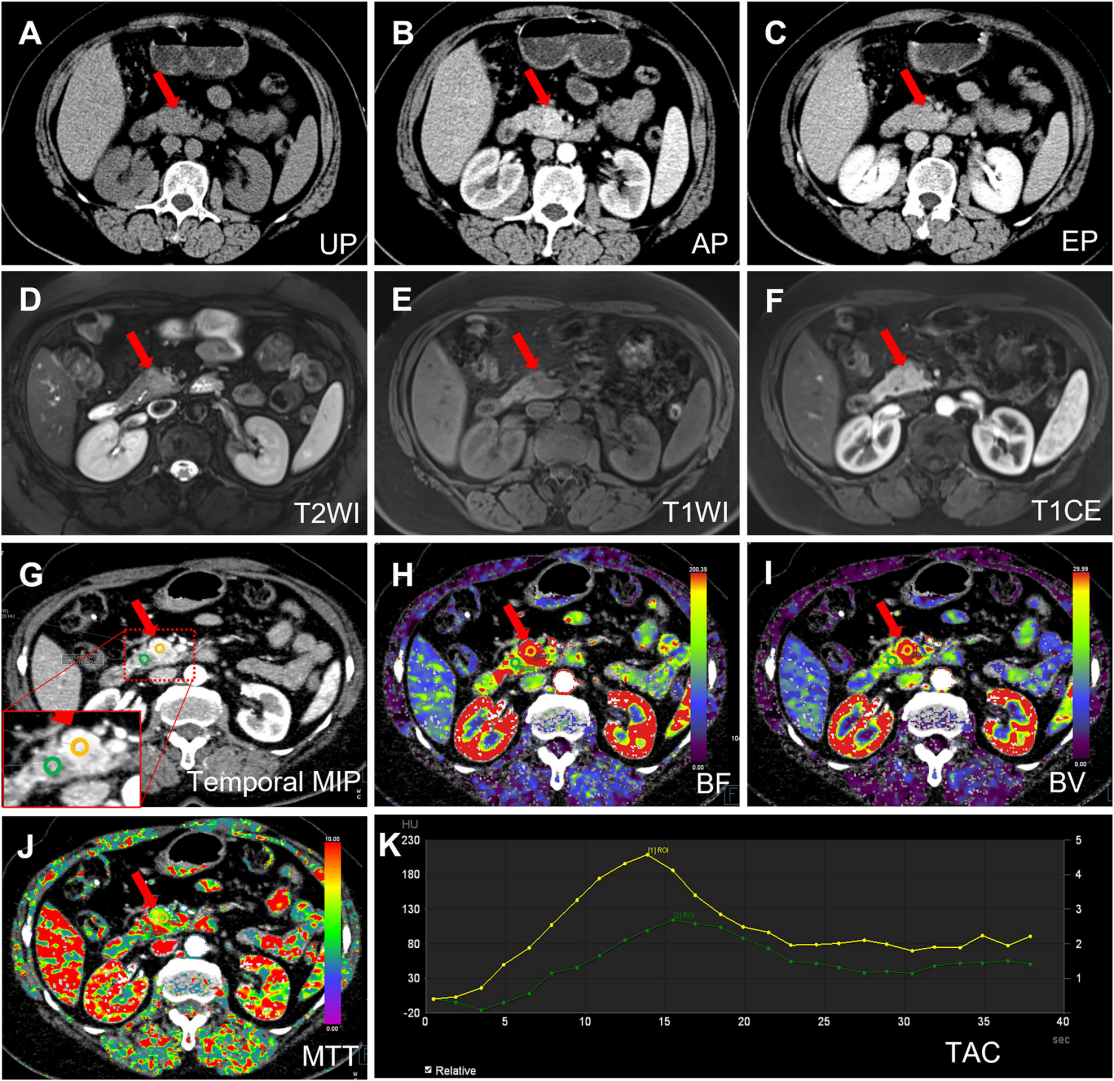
A 65-year-old female (No. 16 in Table S5) was admitted for examination after experiencing recurrent hypoglycemia. The first row shows CECT images, including unenhanced phase (UP) (A), arterial phase (AP) (B), and excretory phase (EP) (C). The second row displays MR images, comprising T2-weighted image (T2WI) (D), T1-weighted image (T1WI) (E), and contrast-enhanced T1-weighted arterial phase image (T1CE) (F). The third and fourth rows present pCTP images, including temporal maximum intensity projection (MIP) (G), color-coded blood flow (BF) (H), color-coded blood volume (I), mean transit time (MTT) (J) maps, and time-attenuation curve (TAC) (K). Yellow circle and curve indicate the ROI of tumor (ROI1) and green circle and curve indicate the ROI of normal pancreatic parenchyma (ROI2). Red arrows indicate the tumor
